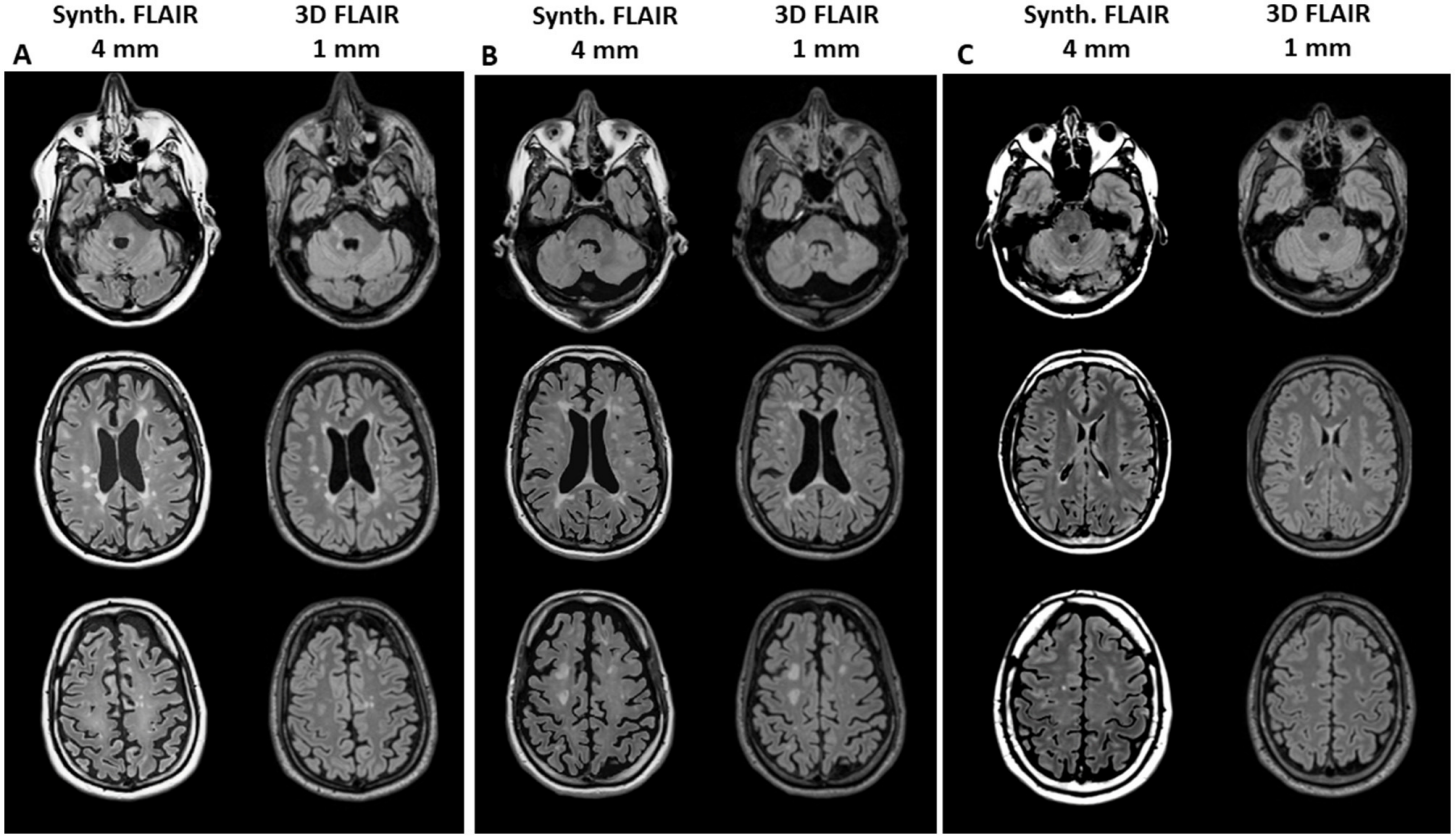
Example images of three patients with high lesion volume [(A) male, age 50 years, SPMS, TLV 28 ml and (B) male, age 38 years, RRMS, TLV 27 ml] or low lesion volume [(C) male, age 30 years, RRMS, TLV 0.5 ml] for visual comparison between synthetic FLAIR images (with 4 mm slice thickness, left column in each block) and the original 3D FLAIR images (1 mm slice thickness, right column). The upper row shows infratentorial sections, the middle row shows ventricular sections, and the lower row shows supraventricular sections.
